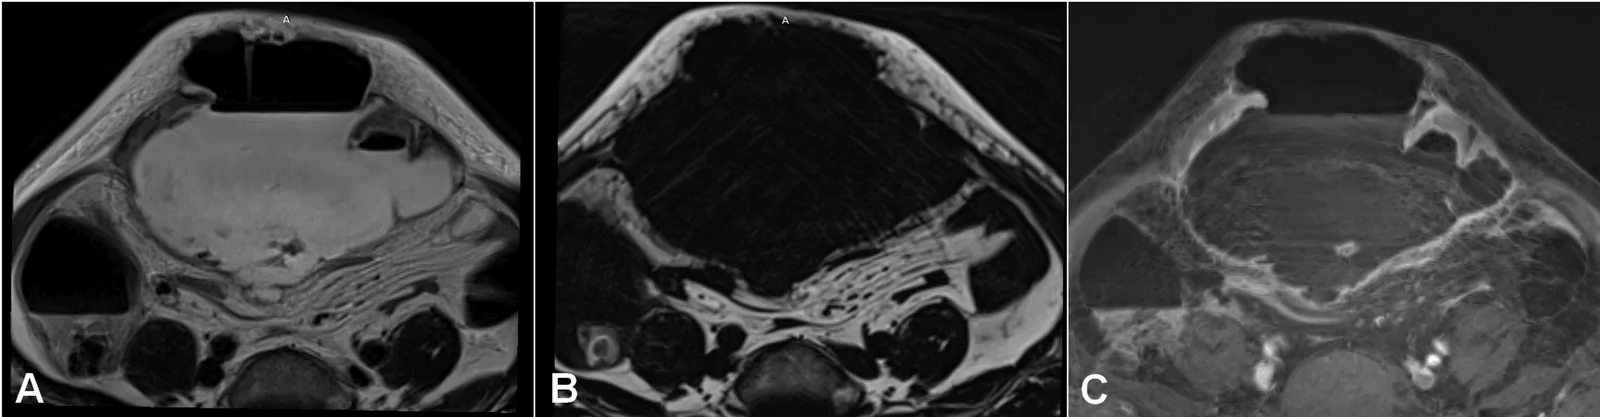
Axial magnetic resonance images showing a large urachal abscess with no signs of malignant degeneration The abscess is seen as a heterogeneous hyperintense signal on T2-weighted image (A), and as a hypointense signal on T1-weighted image (B), with peripheral contrast enhancement (C).
